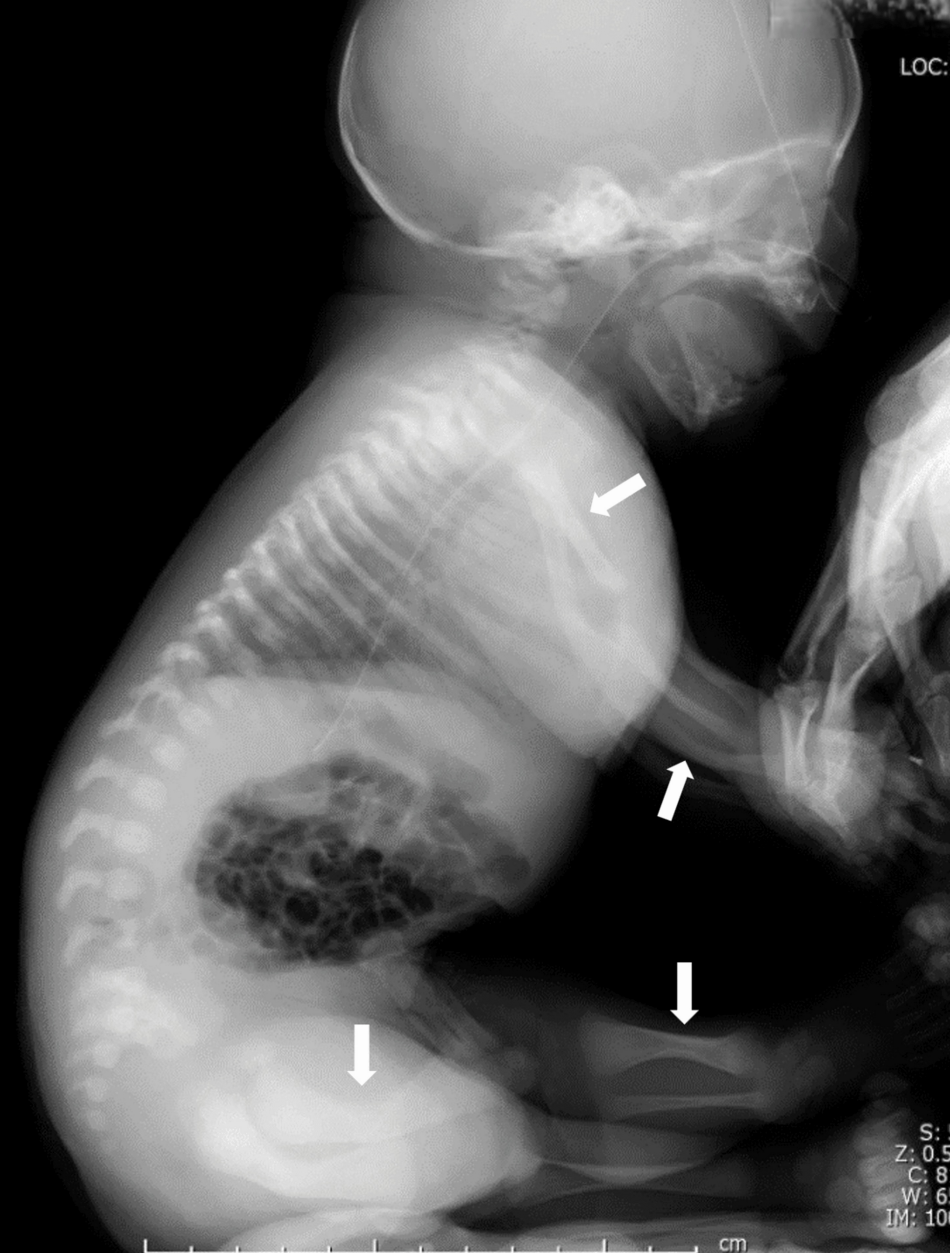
Side view of the neonate's trunk on X-ray Along with the upper arm and forearm, the long bones of the lower leg are also hypoplastic and curved (white arrow). No notable findings were observed in the vertebrae.
